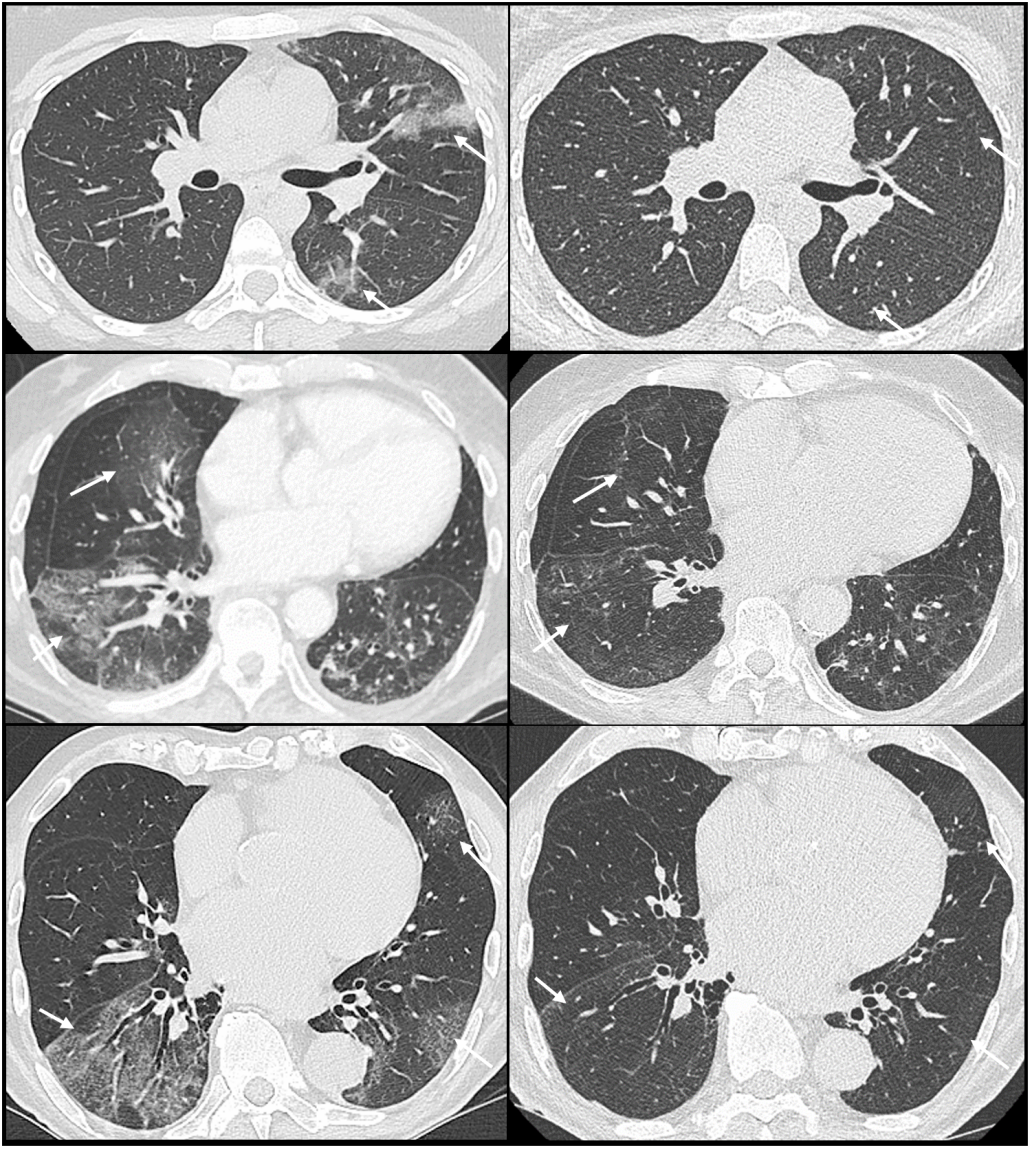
CT scan before (left) and after (right) intravenous immunoglobulin in immunocompromised patients with COVID-19 showing clear reduction in ground-glass opacities (arrows).
